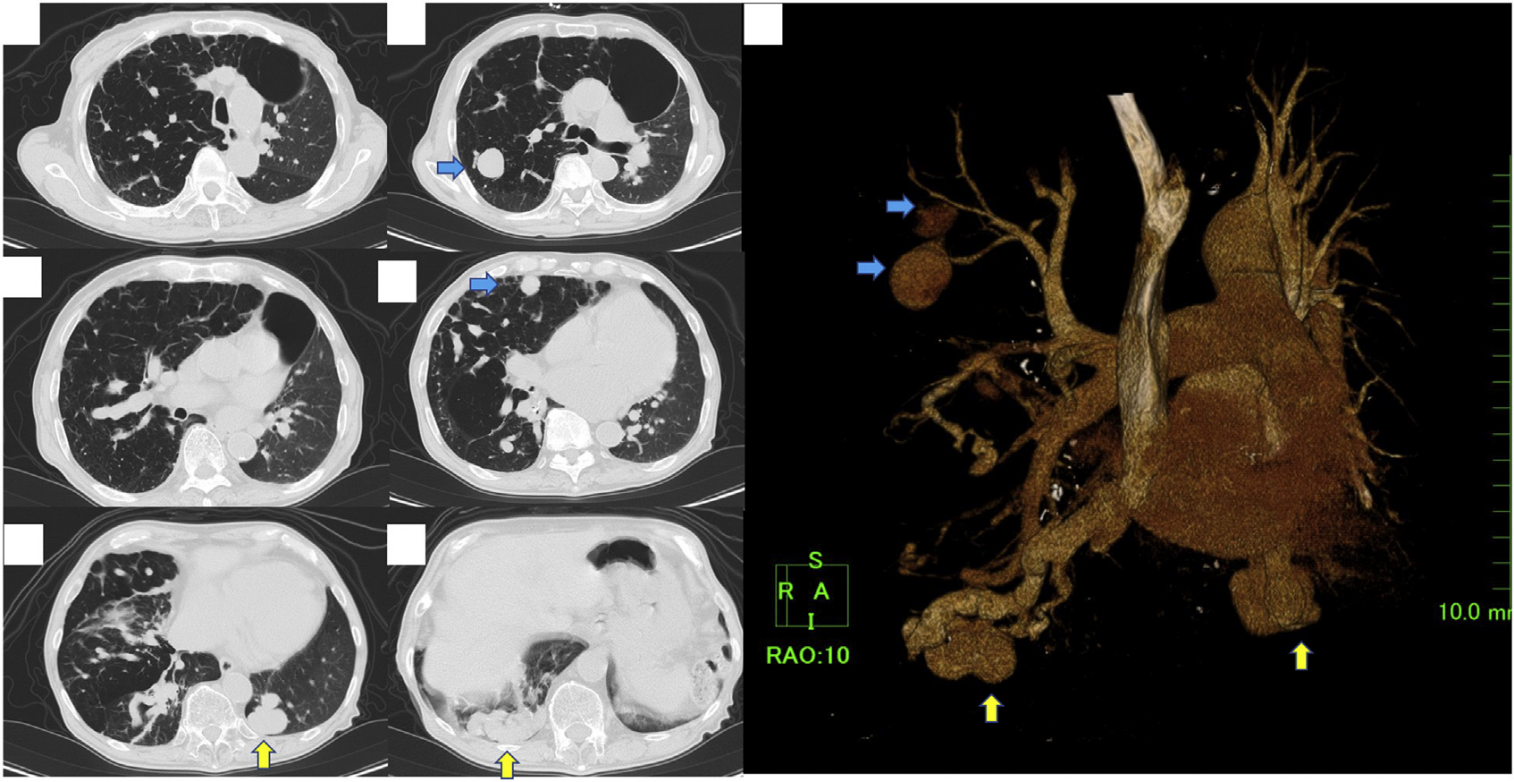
Computed Tomography of the Lung Computed tomography revealed multilobular fistulas (yellow arrows) and aneurysms (blue arrows).
